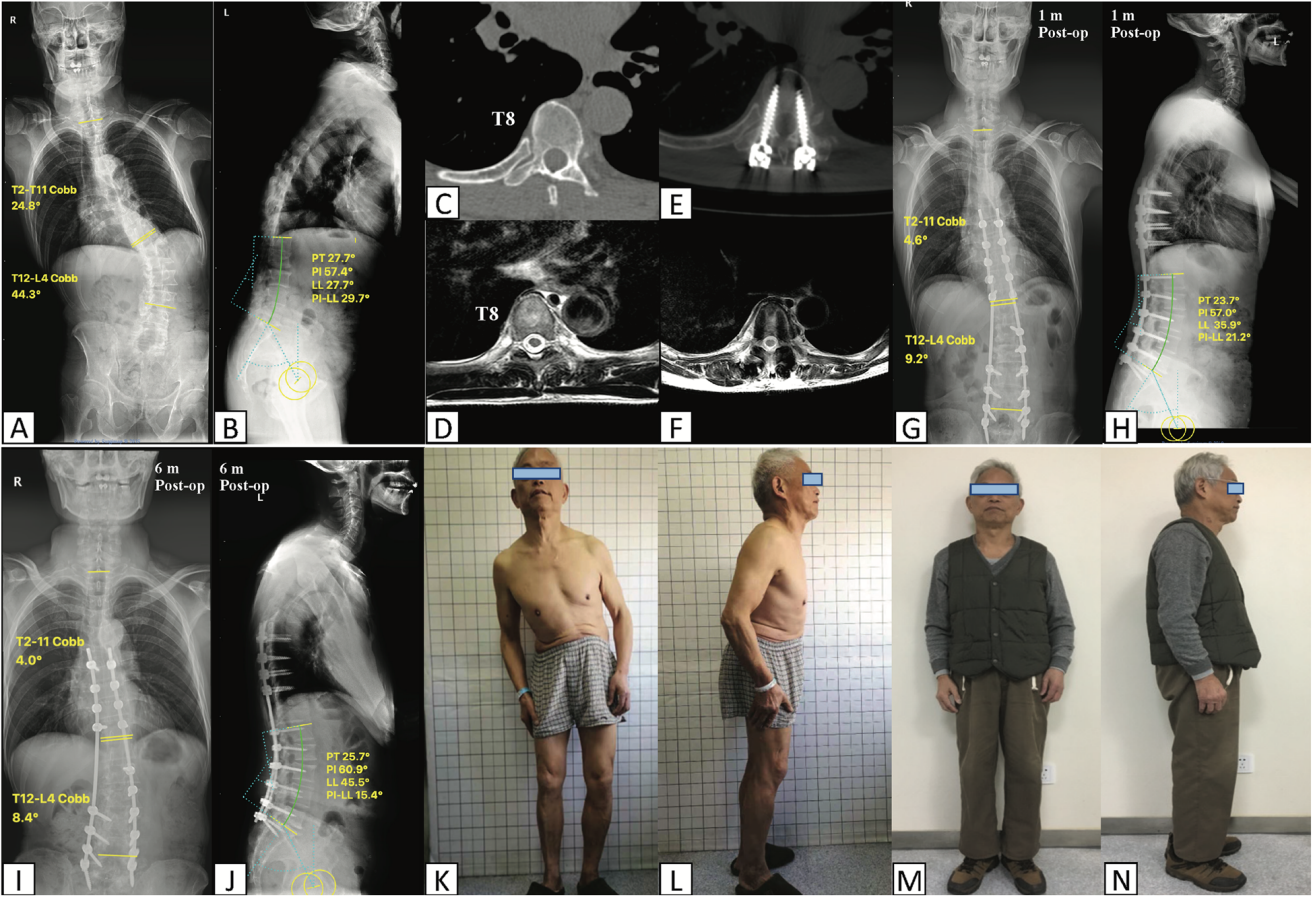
A 74-year-old gentleman with spine deformity for 3 years (K-L). The radiographs demonstrate a lumbar curve of 44.3° and a thoracic curve of 24.8° (a). The sagittal parameters were shown in (b). Postoperative 1 month and 6 months posteroanterior and lateral radiographs demonstrate satisfying correction of degenerative scoliosis (g–j, m, n). c, d Axial plane of pre-op CT and MRI. e, f Post-op CT and MRI revealed satisfying position of T8 pedicle screws (grade A)
